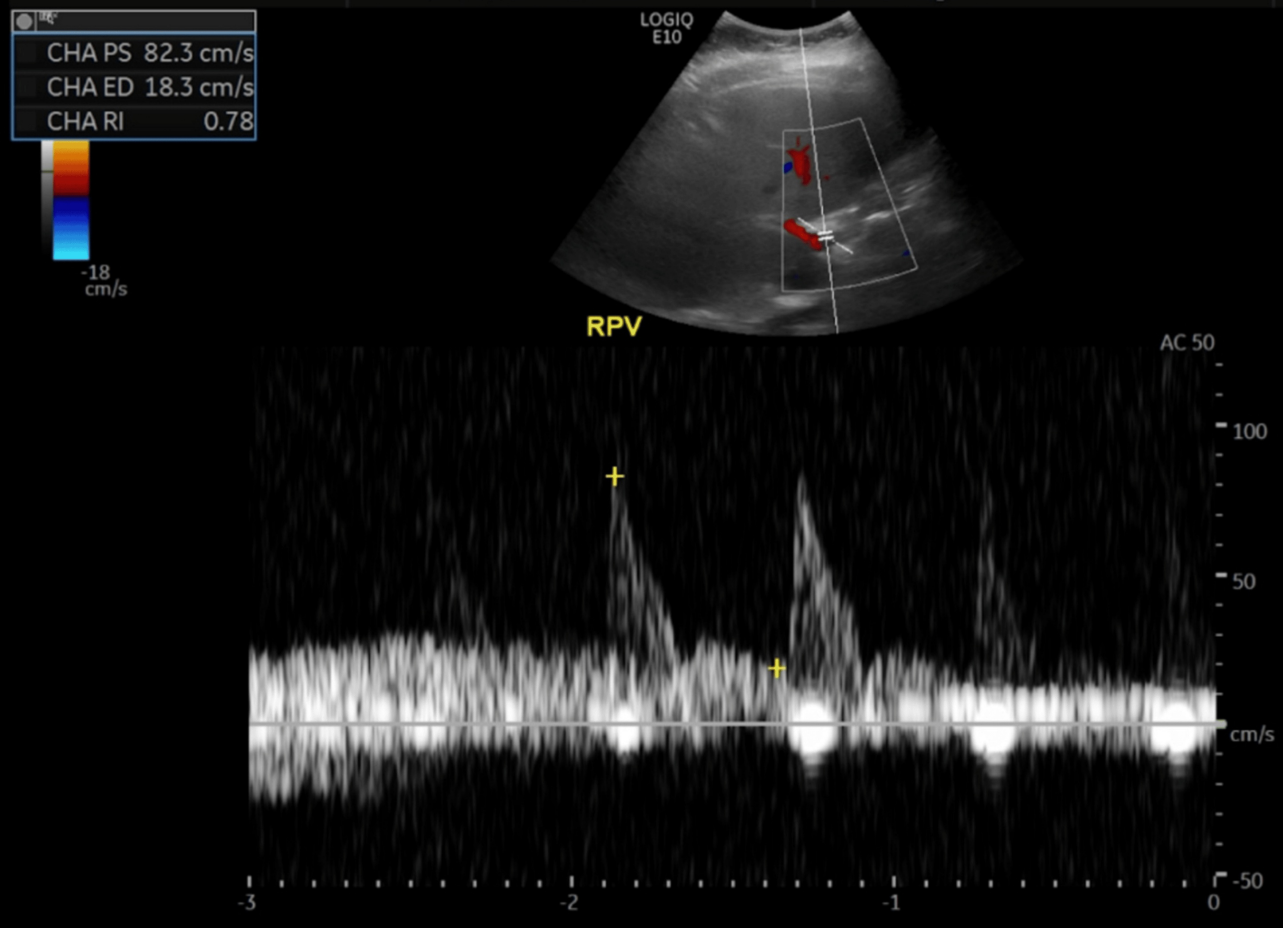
Doppler Ultrasound of the Liver
